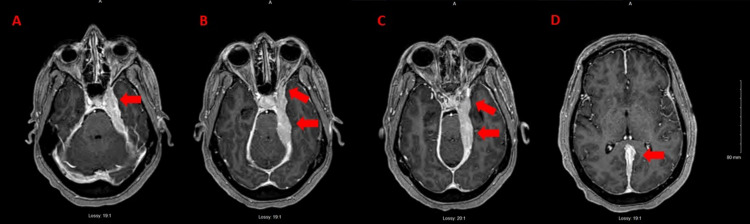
MRI sequences before biopsy of dural lesion. MRI post-axial T1 stealth sequence demonstrating enhancement of cavernous sinus (A), extension into the sella (A and B) and left optic nerve (B), enhancement of the tentorium (B and C), and extension into the falx (D).
